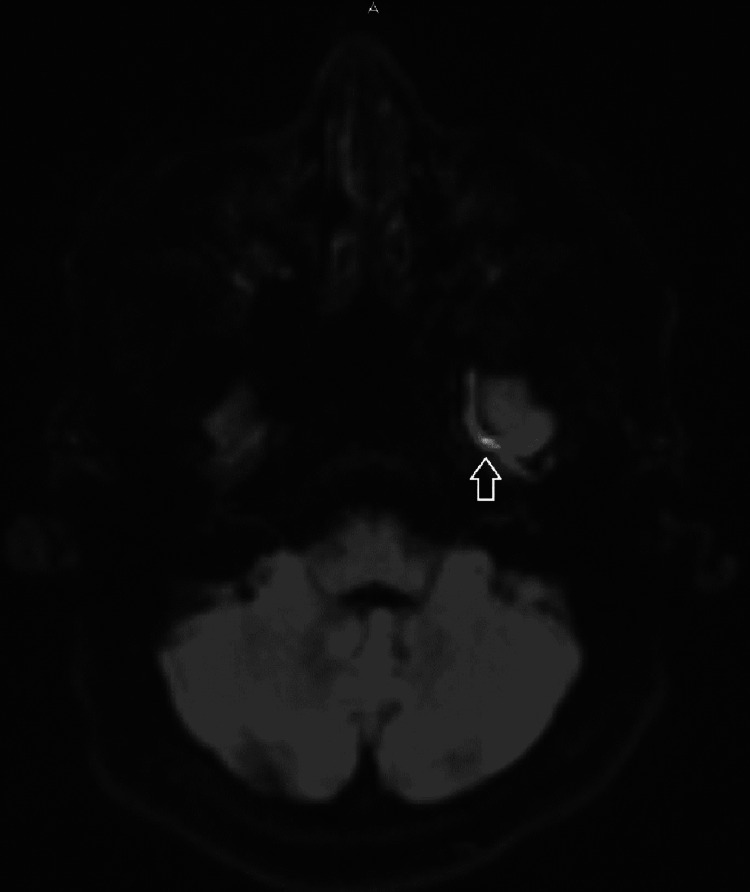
MRI-FLAIR with hyperintensity signal due to cerebral vein thrombosis (arrow) MRI: magnetic resonance imaging; FLAIR: fluid-attenuated inversion recovery
